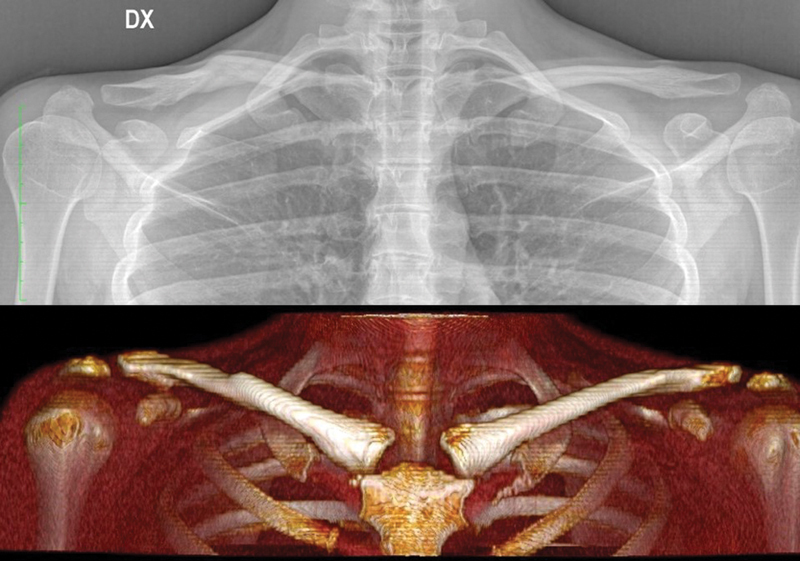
Preoperative X-ray and CT scan 3D reconstruction. 3D, three-dimensional; CT, computed tomography.
